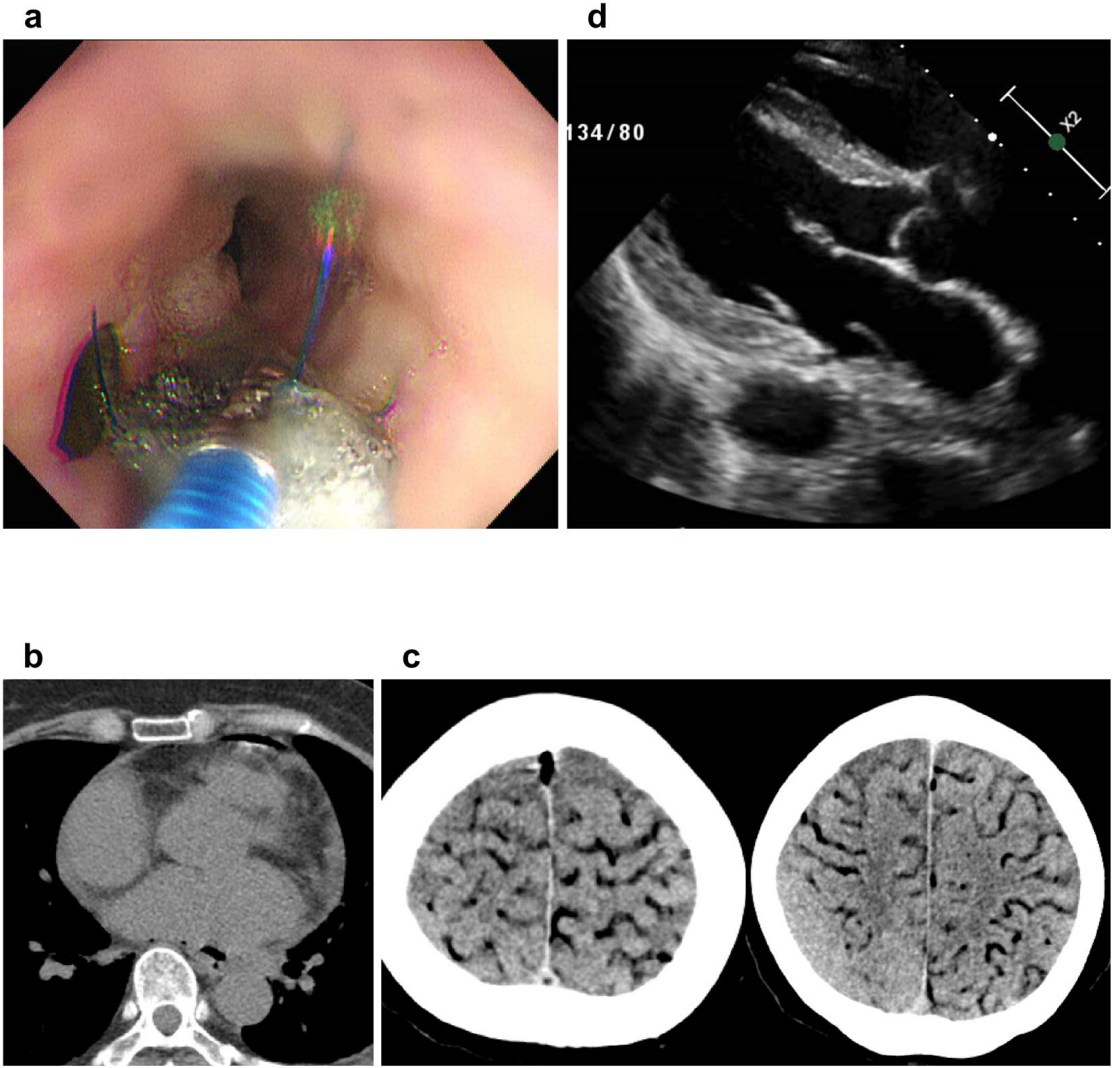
Unsuccessful approaches for atrio-esophageal fistula. (a) Multiple endoscopic repair attempts using sponge material failed to heal atrio-esophageal fistula. (b) Primary repair of the LA from the external side failed to heal LA perforation lesion. Large vegetation originating from LA perforation site is observed in echocardiography. Patient underwent on-pump, open heart redo surgical correction of the LA which was successful. (c and d) The patient suffered massive cerebral air embolism after primary esophageal repair without LA repair. LA: left atrium.
